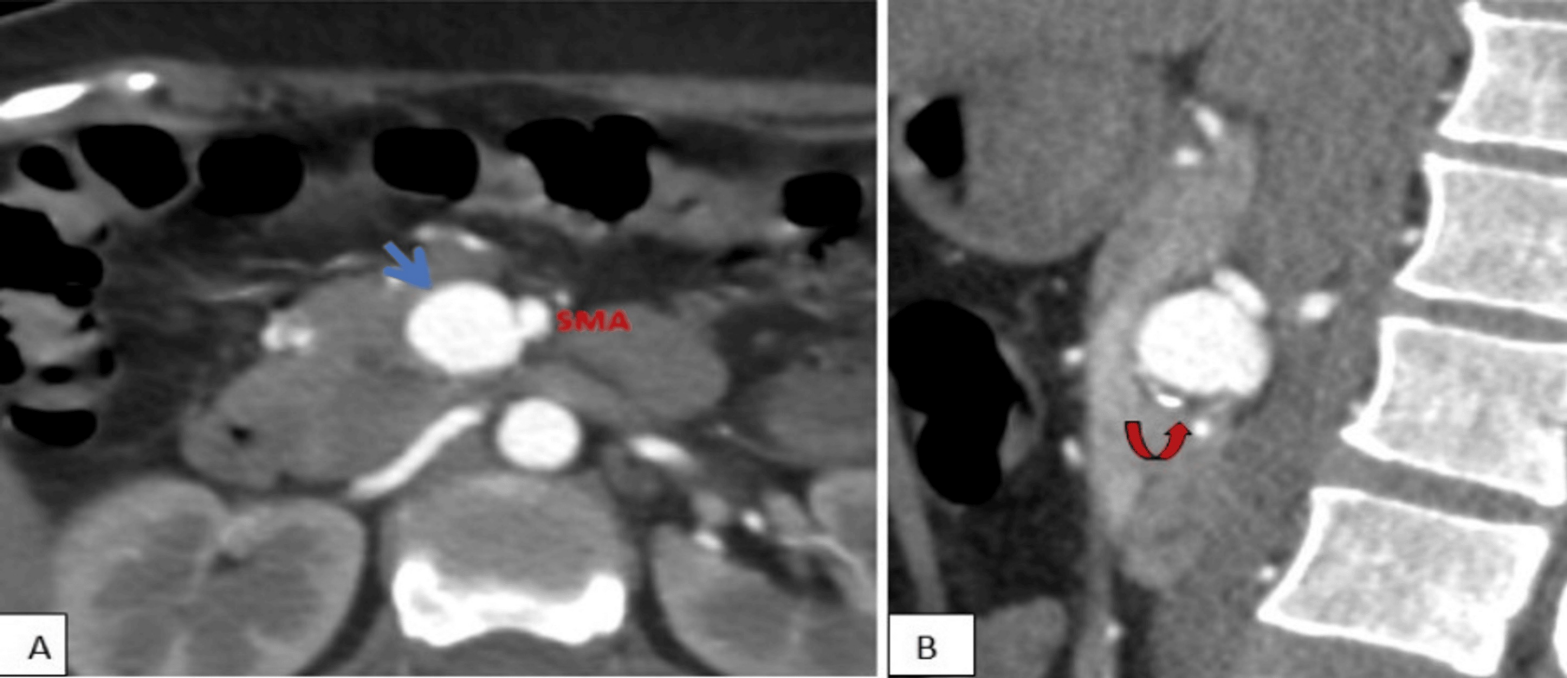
CECT abdomen of a 40-year-old women with pain in abdomen diagnosed with AOB aneurysm (Case 1) (A) Axial section in the arterial phase showed the saccular AOB aneurysm (blue arrow )in close relation with the SMA at the peripancreatic region; (B) Reformatted sagittal view in the arterial phase shows eccentric intramural thrombus and focal wall calcifications of the AOB aneurysm (curved arrow). CECT: contrast-enhanced computed tomography; AOB: Arc of Buhler; SMA: superior mesenteric artery
